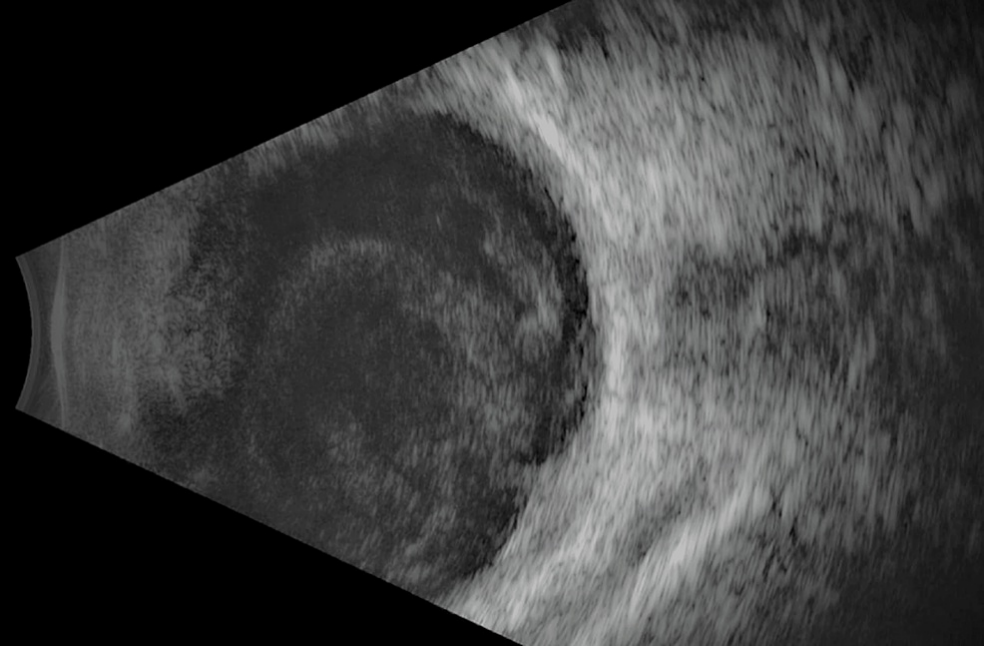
Ultrasound B-scan findings The image showed scleral thickening with the presence of vitreous opacities
